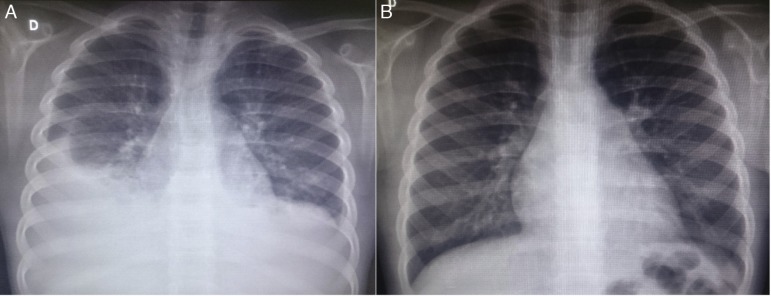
Chest radiography images of the patient in the posteroanterior view; A-at patient admission, costophrenic sinus obliteration is observed bilaterally, with pleuropulmonary opacity to the right; B-six months after discharge, during an outpatient consultation, the radiography shows no alterations.
